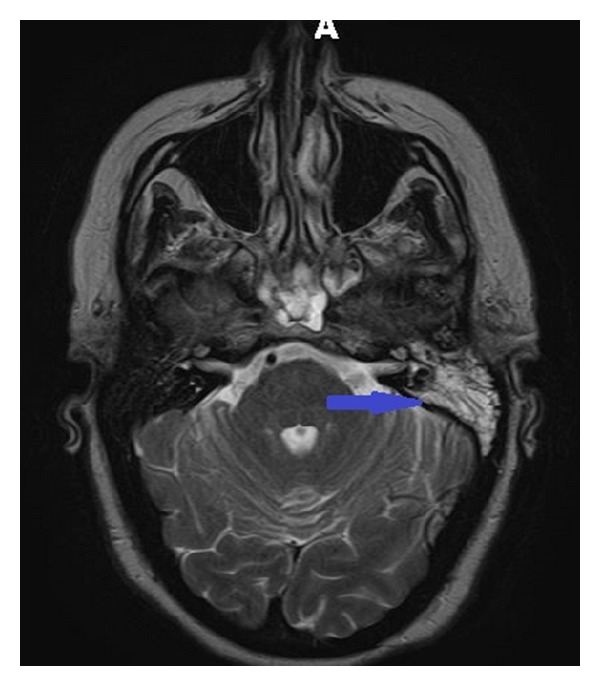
The MRI shows enhancement in the temporal bone region equivocal for inflammation and infection (blue arrow).
